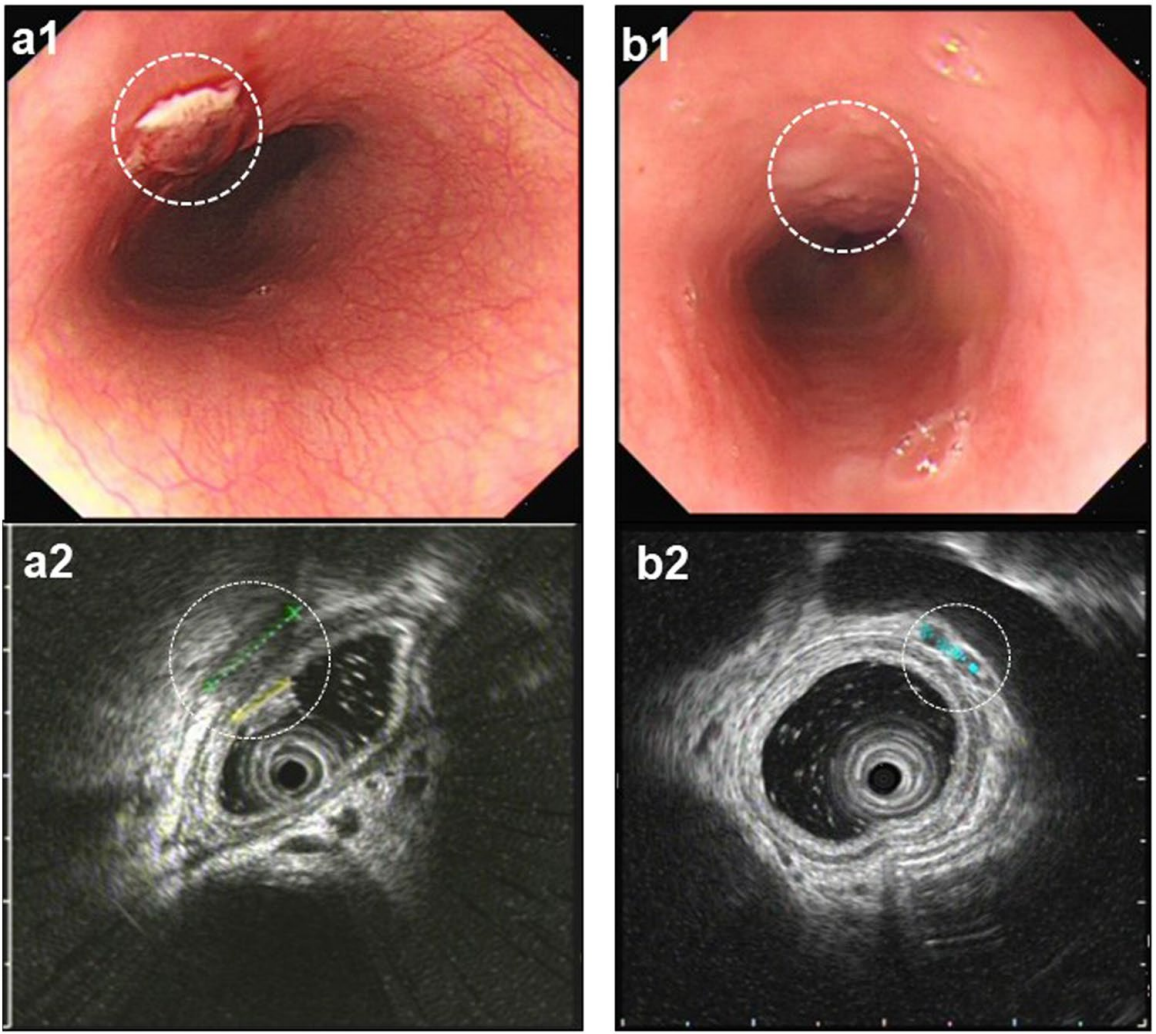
Esophageal injury post ablation shown by endoscopic ultrasonography. Gastroscopy revealed esophageal ulcer (a1, white dot circle) 2 days after radiofrequency ablation. Ultrasonography displayed the thickening and loss of the submucous layers architecture (a2, white dot circle). The esophagus recovered with normal architecture 10 days later (b1, b2, white dot circle).
